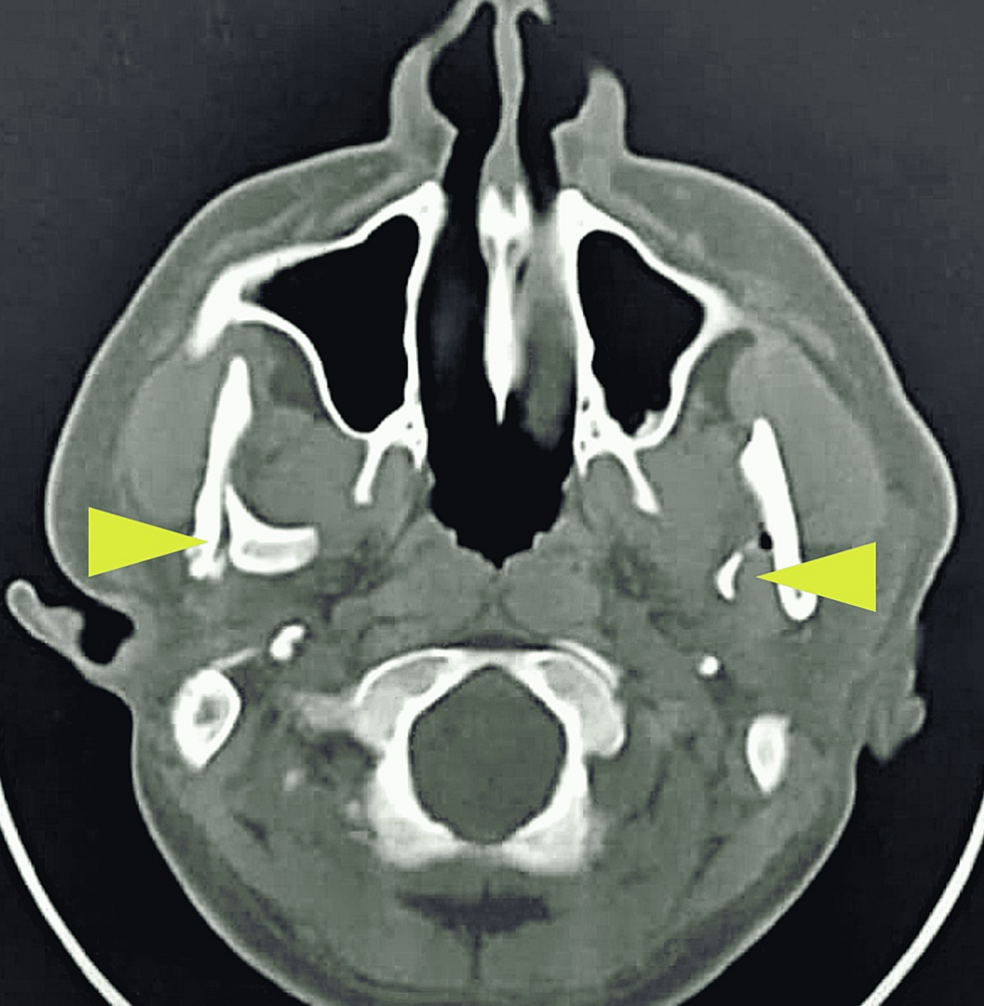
Axial section of a computed tomography scan revealing bilateral medial dislocation of mandibular condyles (arrow)
